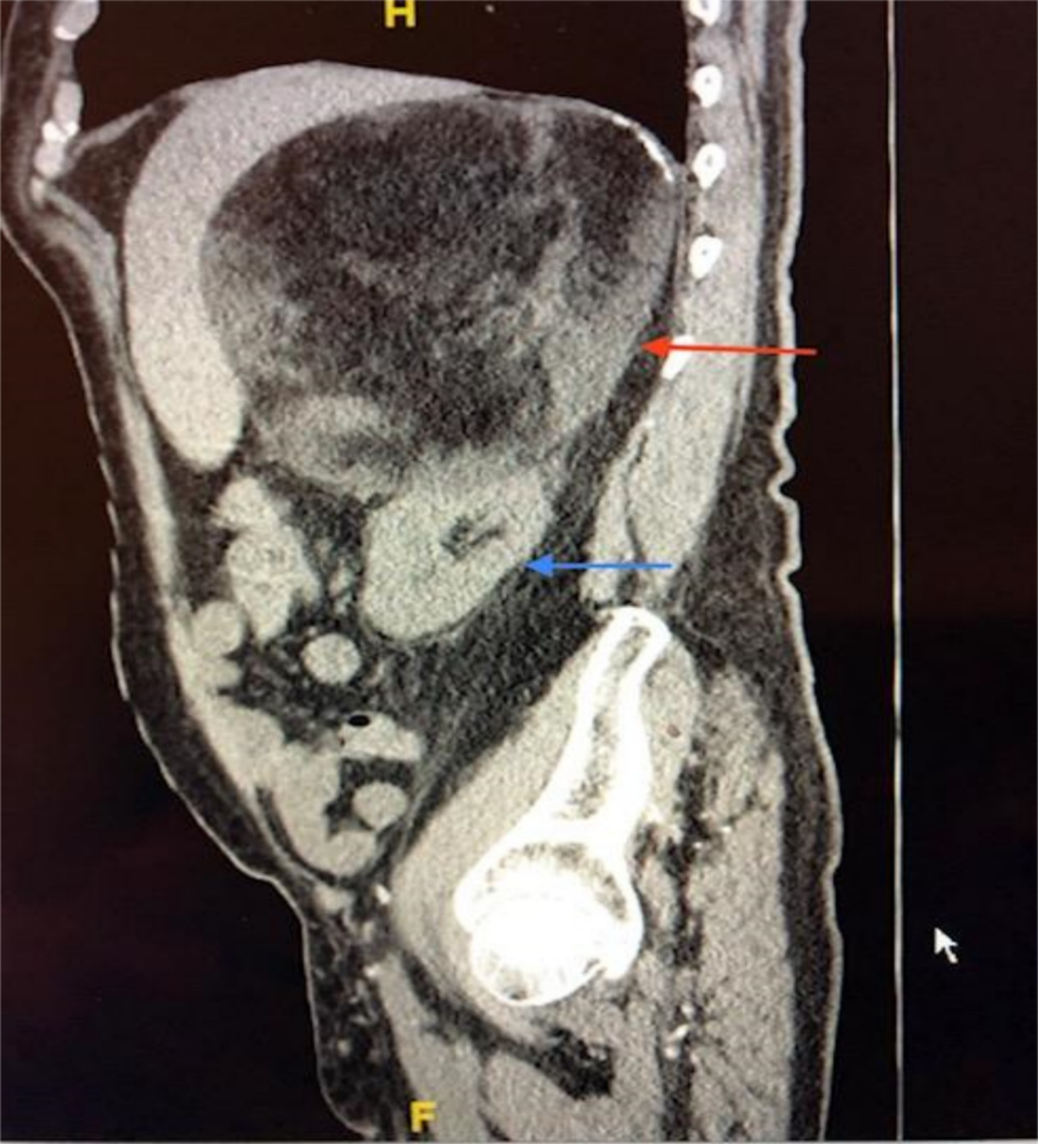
Computed tomography of the abdomen and pelvis Adrenal mass (red arrow); Kidney (blue arrow)
